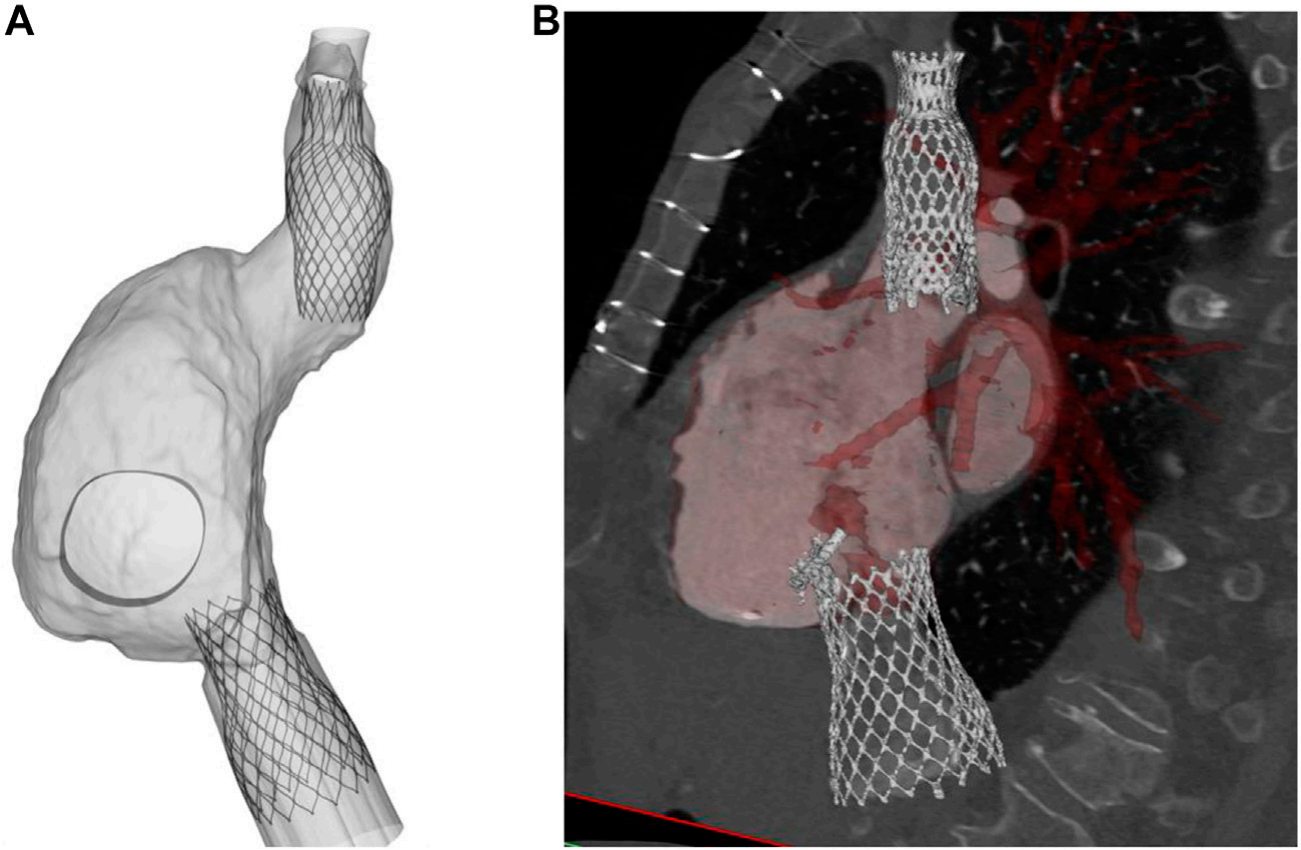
(A) deformed shape of implanted TricValve system for Case B and (B) CT scan after TricValve implantation.
